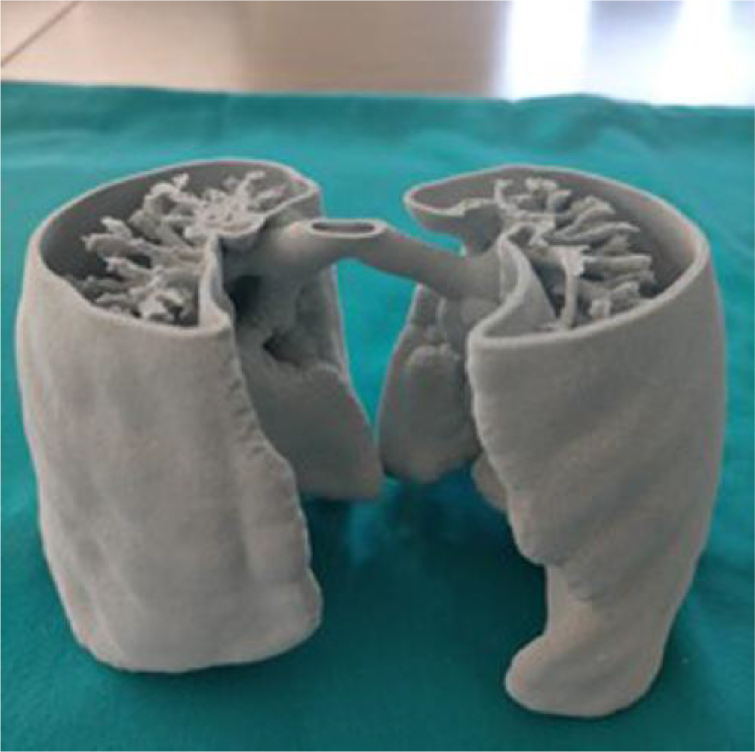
3D printing from a CT scan of lungs of healthy children. The irregular geometric figure can be appreciated.
